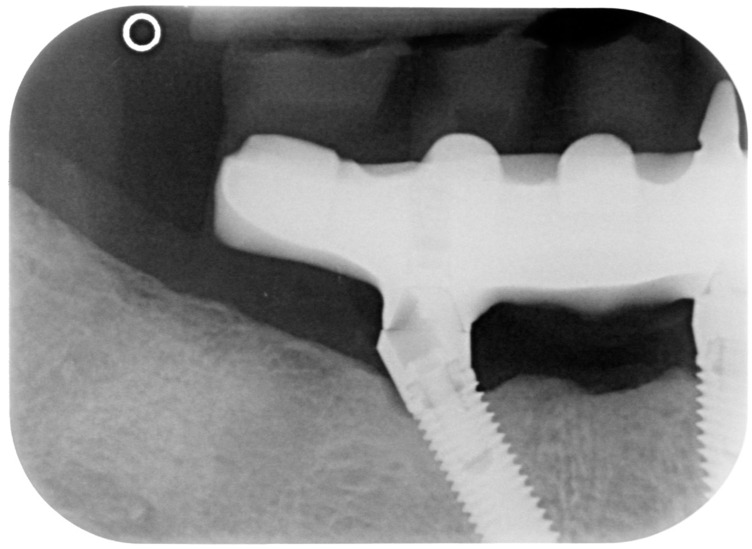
X-ray of 4.5 dental implant in patient in 6 months after treatment.
